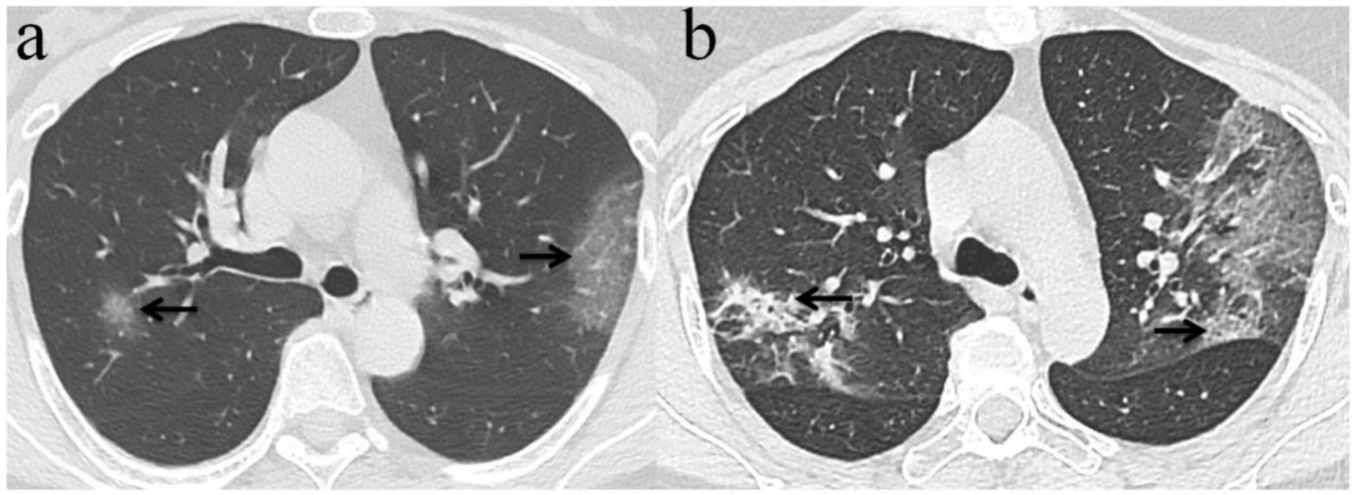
Chest CT imaging findings of COVID-19 pneumonia in a 56-year-old female patient presenting with fever (range:38.1-39℃) for five days. (a) GGO was presented in the upper lobes of bilateral lung with initial negative RT-PCR (arrow); (b) 6 days later, the upper lobes of bilateral lung show extensive consolidation and GGO while RT-PCR test turned positive. (arrow).
